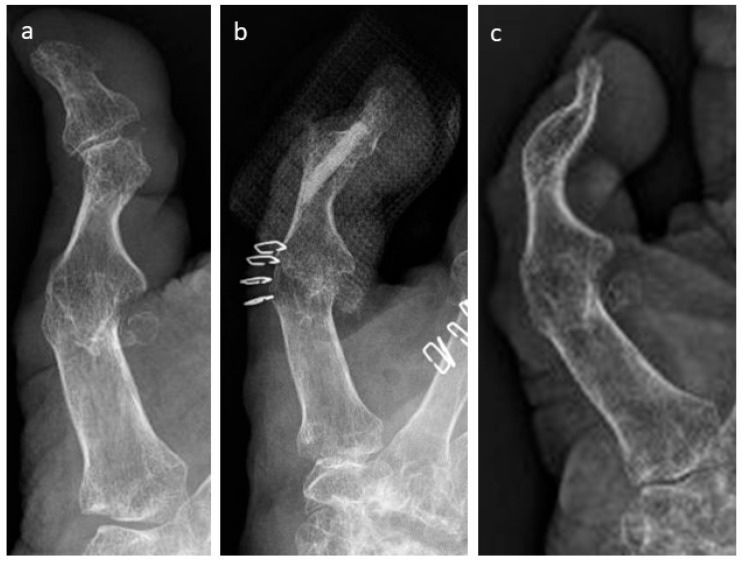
A failed thumb IP-arthrodesis of a patient with chronic polyarthritis is depicted. (a): presurgical X-ray; (b): the patient was treated with one Shark Screw^®^, and union was recorded after 12 weeks; (c): the Shark Screw^®^ was totally remodelled into the host bone after 21 months.
